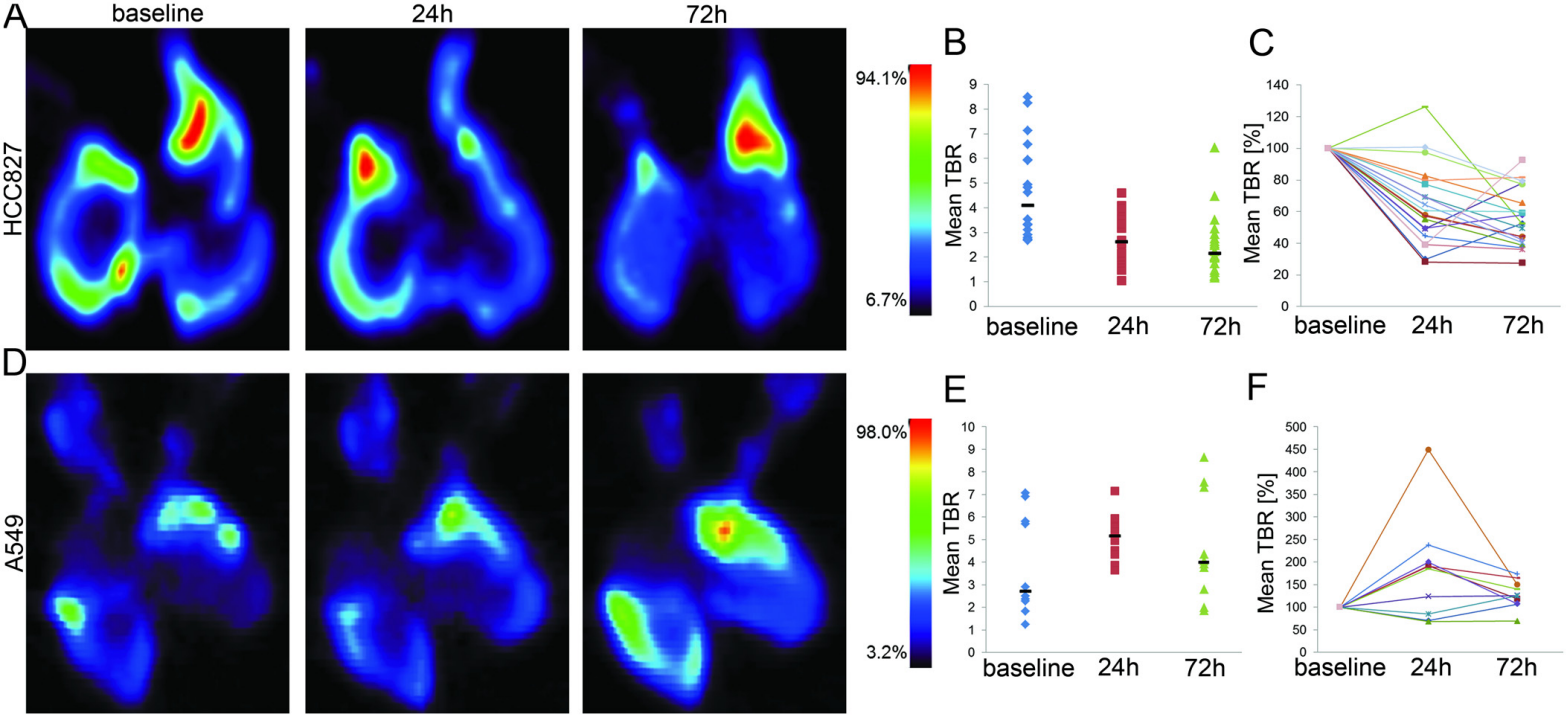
Monitoring of treatment response to targeted therapy with FDG-PET in lung tumor models. Lungs recellularized with HCC827 (top panel, A) or A549 (bottom panel, D) were incubated with ^18^F-FDG-PET for 60 min and imaged using a μPET-scanner. Coronal views of exemplary lung scaffold cultures (n = 3) at baseline and following 24 h or 72 h treatment with gefitinib are shown. 3D regions of interest were defined at individual tumor nodules or scaffold tissue and mean tumor-to-background ratios (TBR) were calculated (B, E). Horizontal bars depict median values for the mean TBRs of individual nodules at baseline, 24 h and 72 h. (C, F) Relative change of tracer uptake in individual tumor clusters compared to ^18^F-FDG-PET-intensity at baseline is shown. Intensity of tracer uptake decreased over the course of 72 h treatment with gefitinib in HCC827-, while no dramatic effect was detected in A549-seeded lungs.
